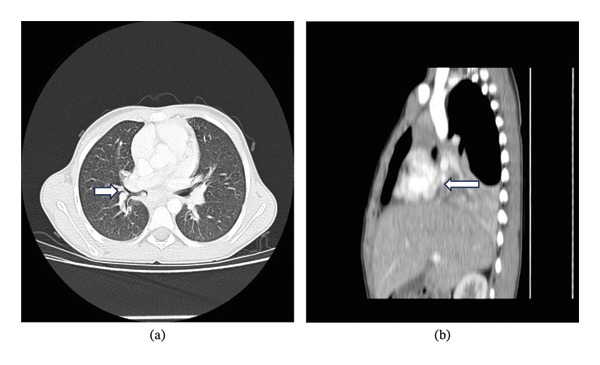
(a) Axial CT demonstrating heterogeneous content protruding into the right main bronchus, as well as the intermedius bronchus. (b) Sagittal CT demonstrating an atelectasis of the entire lower lung lobe on the right and the lateral segment of the middle lobe. CT: computerized tomography.
